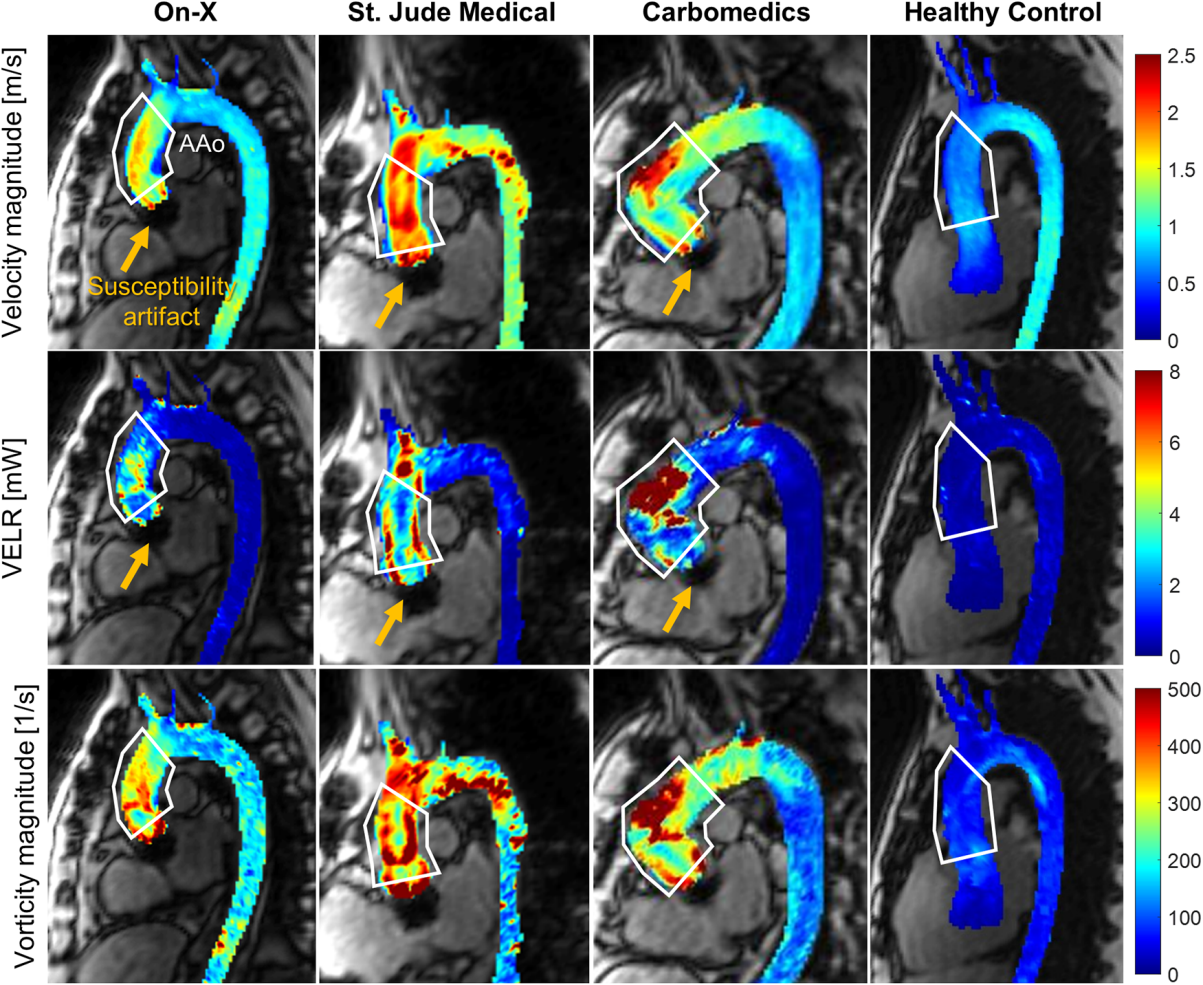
Example images showing the peak systolic aortic flow field quantified by 4D flow MRI in patients with the on-X aortic valve conduit (column 1), St. Jude Medical (column 2), Carbomedics (column 3), and healthy subjects (column 4). The velocity magnitude (row 1) and vorticity magnitude (row 3) are shown as the maximum intensity projection, and VELR (row 2) indicates the viscous energy loss rate. The white polygons indicate the ascending aortic region of interest. Note that the yellow arrows indicate the region of susceptibility artifact due to the presence of a mechanical aortic valve. The temporal evolution of 3D aortic flow in the subjects presented in this figure is visualized using pathlines and is available as Supplementary Videos.
